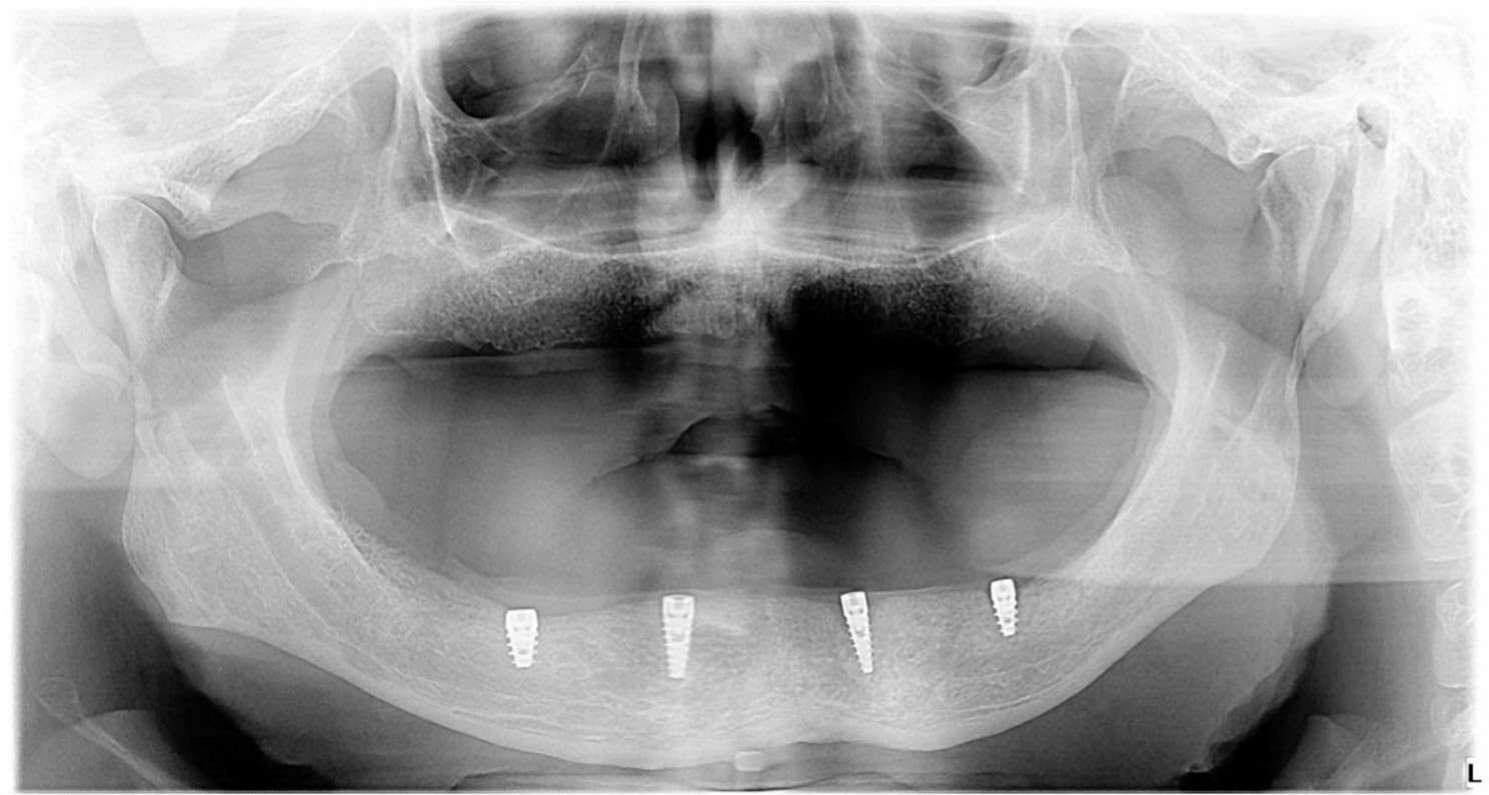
Panoramic x-ray after implant insertion
